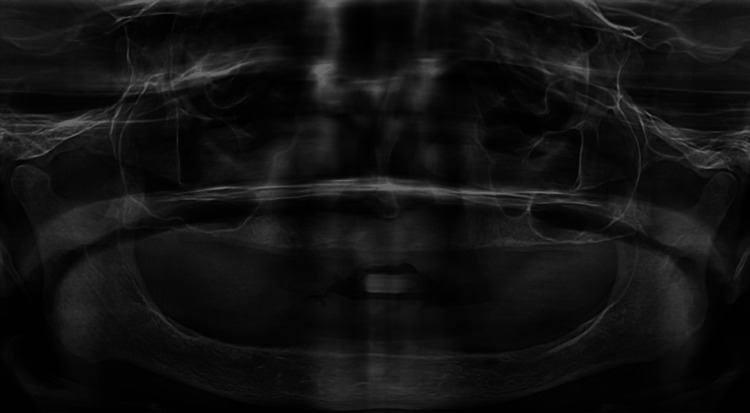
Pre-operative orthopantomogram
